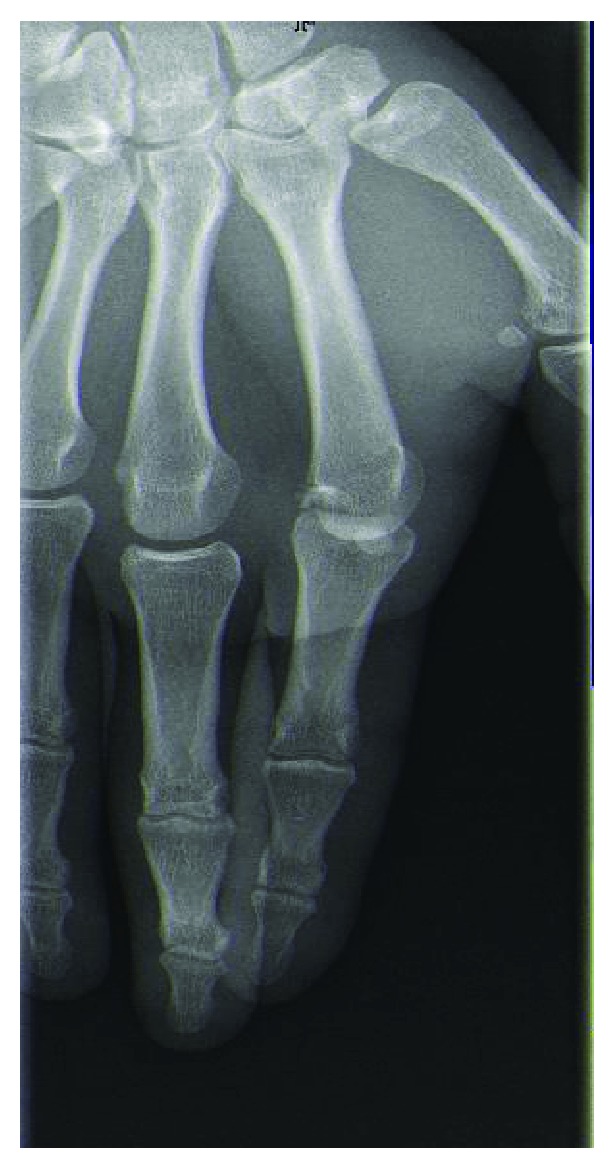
X-Ray (AP view) showing MCP dislocation of the index finger.
